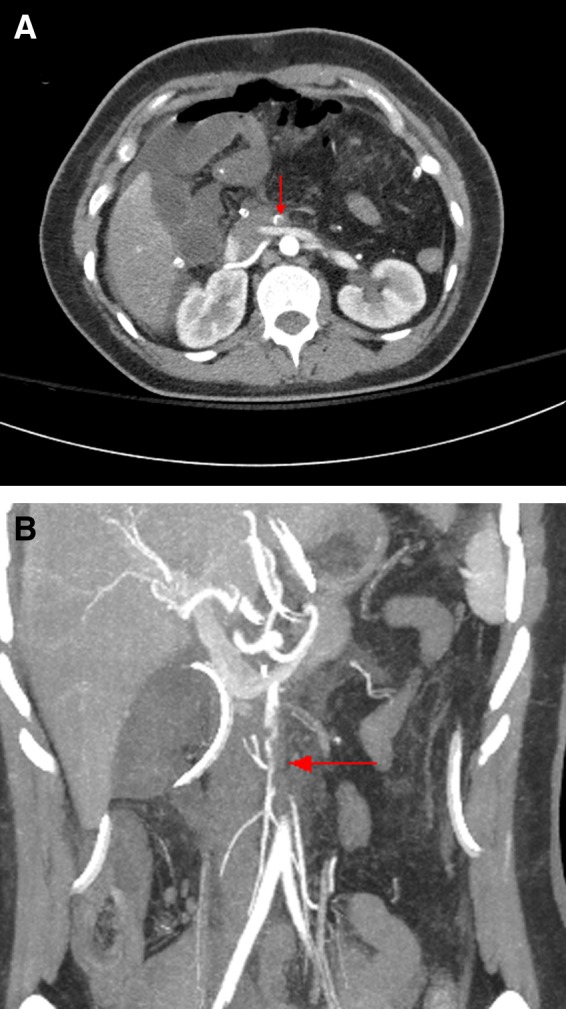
(A, B) Contrast-enhanced abdominal CT scan on postoperative day 1. A = axial. B = coronal. The images reveal an intraluminal thrombus (*red arrow*) in the proximal SMA ∼1.5 cm from its aortic origin, causing near complete occlusion of the SMA.
